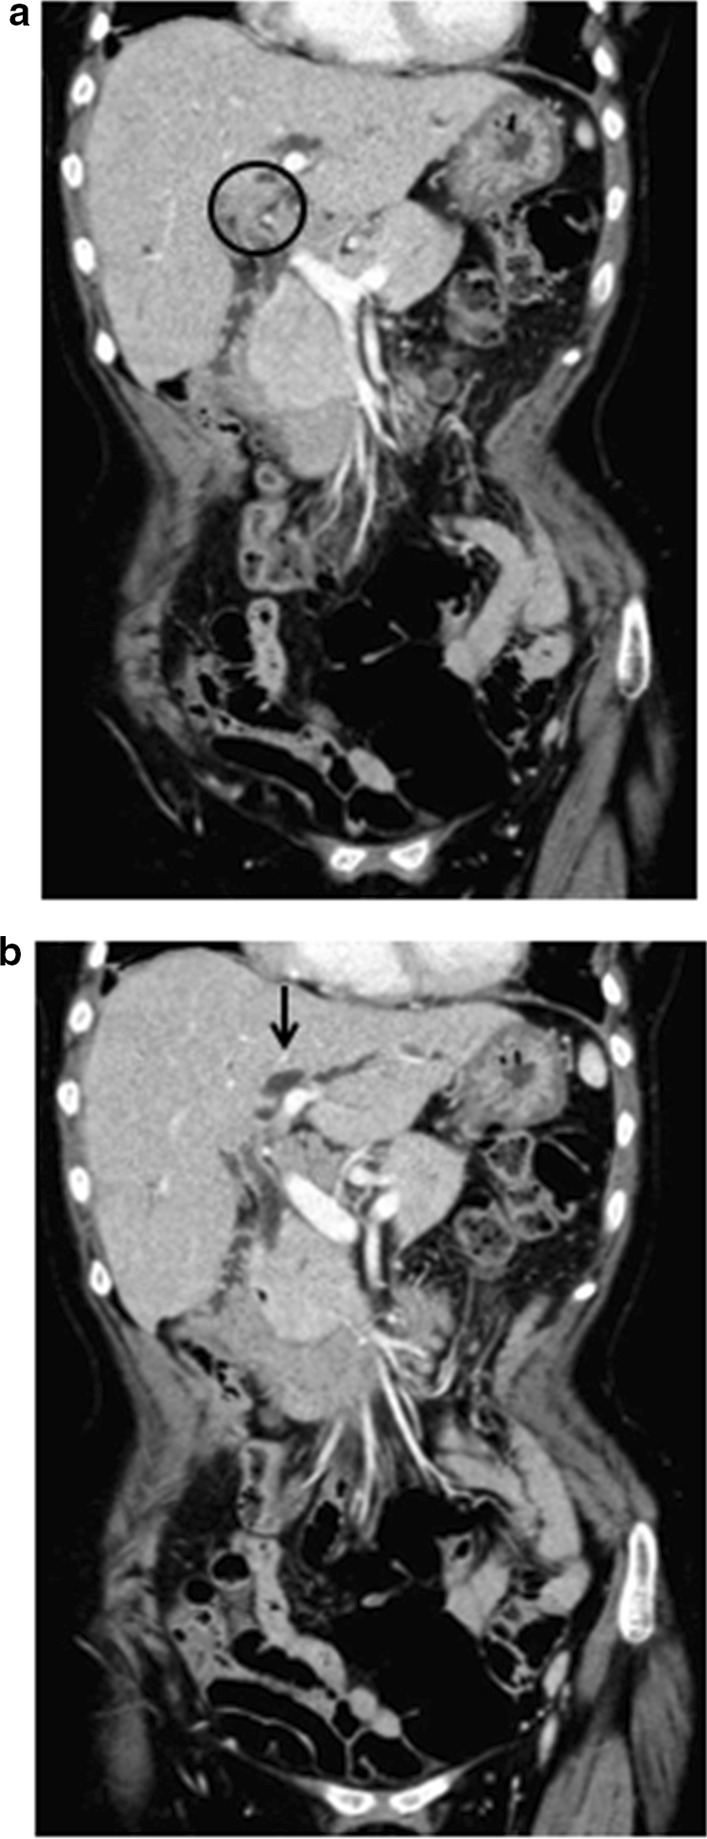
Abdominal computed tomography. a Solid mass in the hilar bile duct (circle). b Slight dilatation of intrahepatic bile ducts in the left lobe (arrow)
